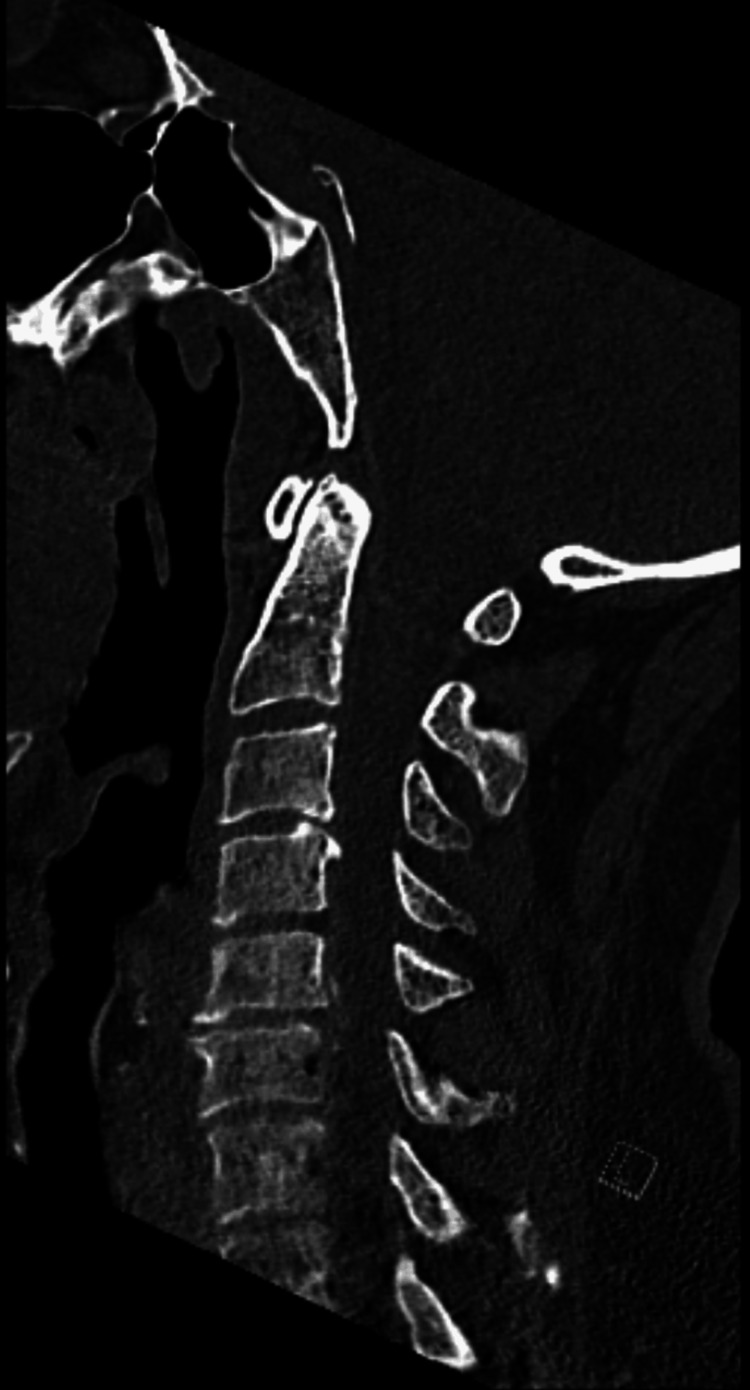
CT cervical spine obtained at admission with no evidence of cervical fracture or dislocation
